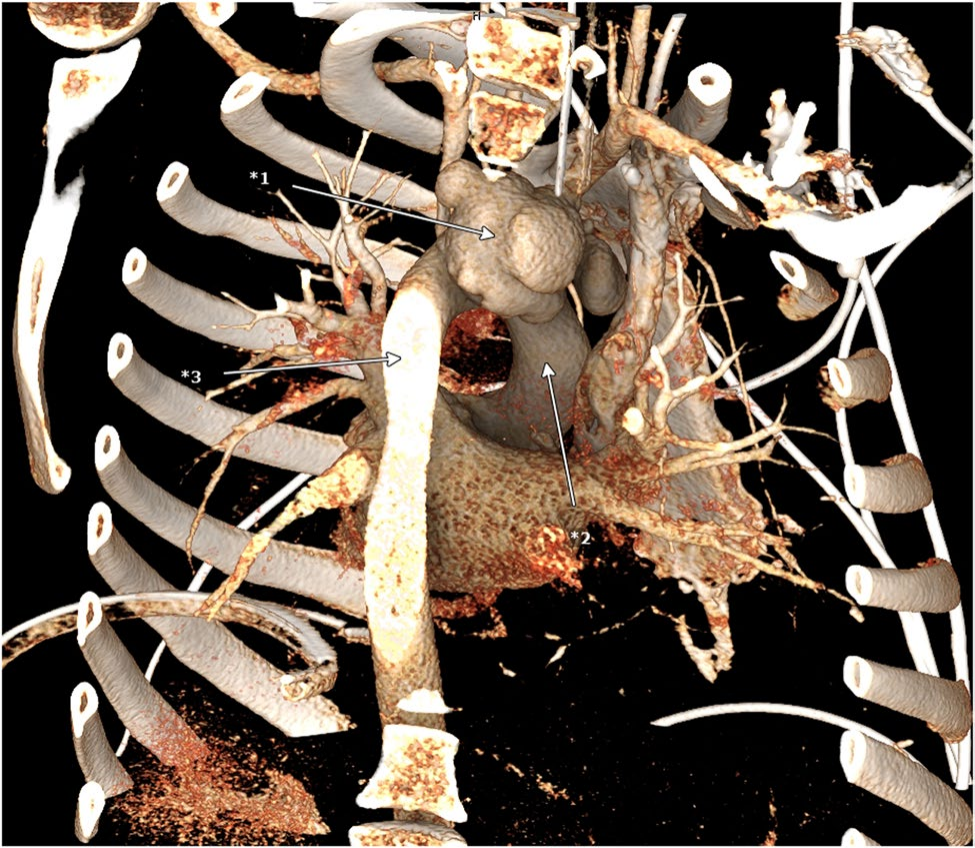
3D reconstruction of the CT scan, view from dorso-lateral, *1 pseudoaneurysm, *2 aortic arch, *3 descending aorta
